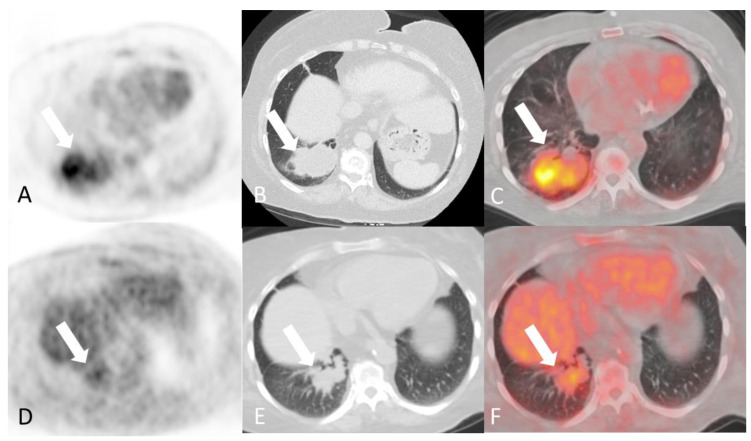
A 50-year-old woman with known granulomatosis with polyangiitis. Axial images of F-18 FDG PET/CT at time of presentation (A–C) demonstrating a tracer avid mass like consolidative opacity in the basal segments of the lower lobe of the right lung (arrows). Axial images of subsequent F-18 FDG PET/CT approximately 6 months after initiation of immunosuppressive therapy (D–F) demonstrating known right lung consolidation is smaller and significantly less tracer avid (arrows).
